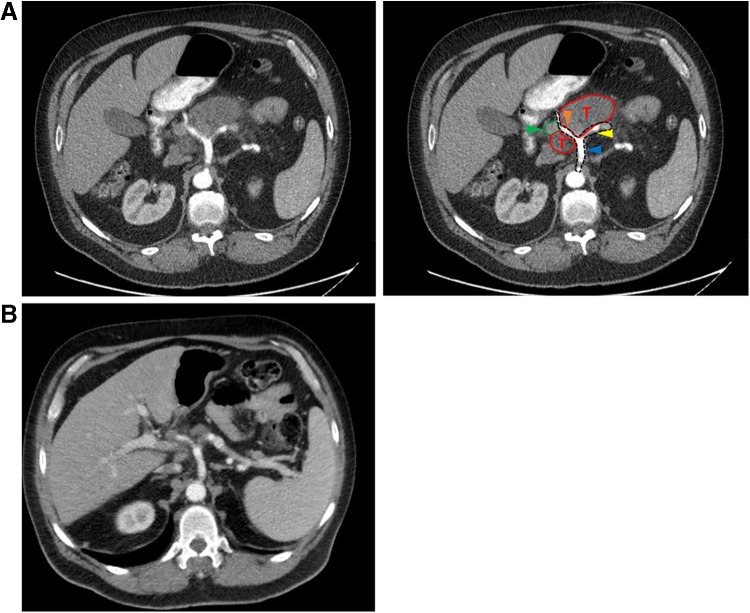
Computed tomography scans of the patient's abdomen, (A) before and (B) after nine cycles of combined chemotherapy. Blue arrowhead = celiac trunk. Yellow arrowhead = splenic artery. Orange arrowhead = common hepatic artery. Green arrowhead = portal vein. T, tumor.
